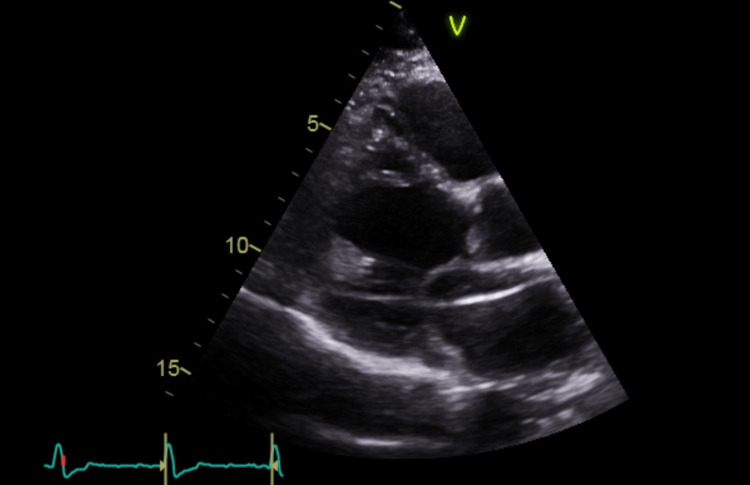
PLAX TTE view showing the lead in the LA going across the mitral valve into the left ventricle PLAX: Parasternal long axis; TTE: transthoracic echocardiogram; LA: left atrium
